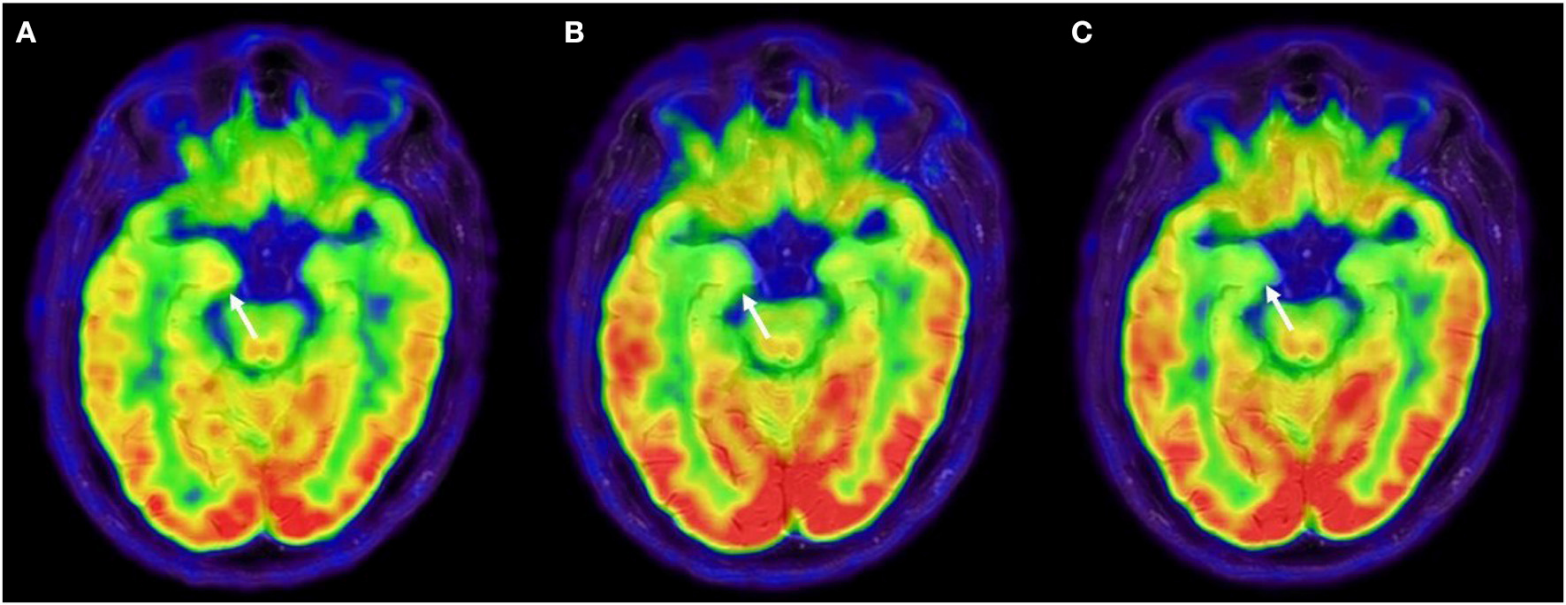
18F-FDG-PET evolution. (A) 18F-FDG-PET after first-line immune therapy showing moderate right amygdala hypermetabolism and bilateral temporomesial, frontoanterior and frontomesial hypometabolism, suggestive for persistence of disease activity. (B) 18F-FDG-PET was repeated after 3 months from rituximab, showing an attenuation of amygdala hypermetabolism and at 10 months from rituximab (C), showing a complete regression of the amygdala hypermetabolism. 18F-FDG-PET, 18F-fluoridesoxyglucose-positron emission tomography.
